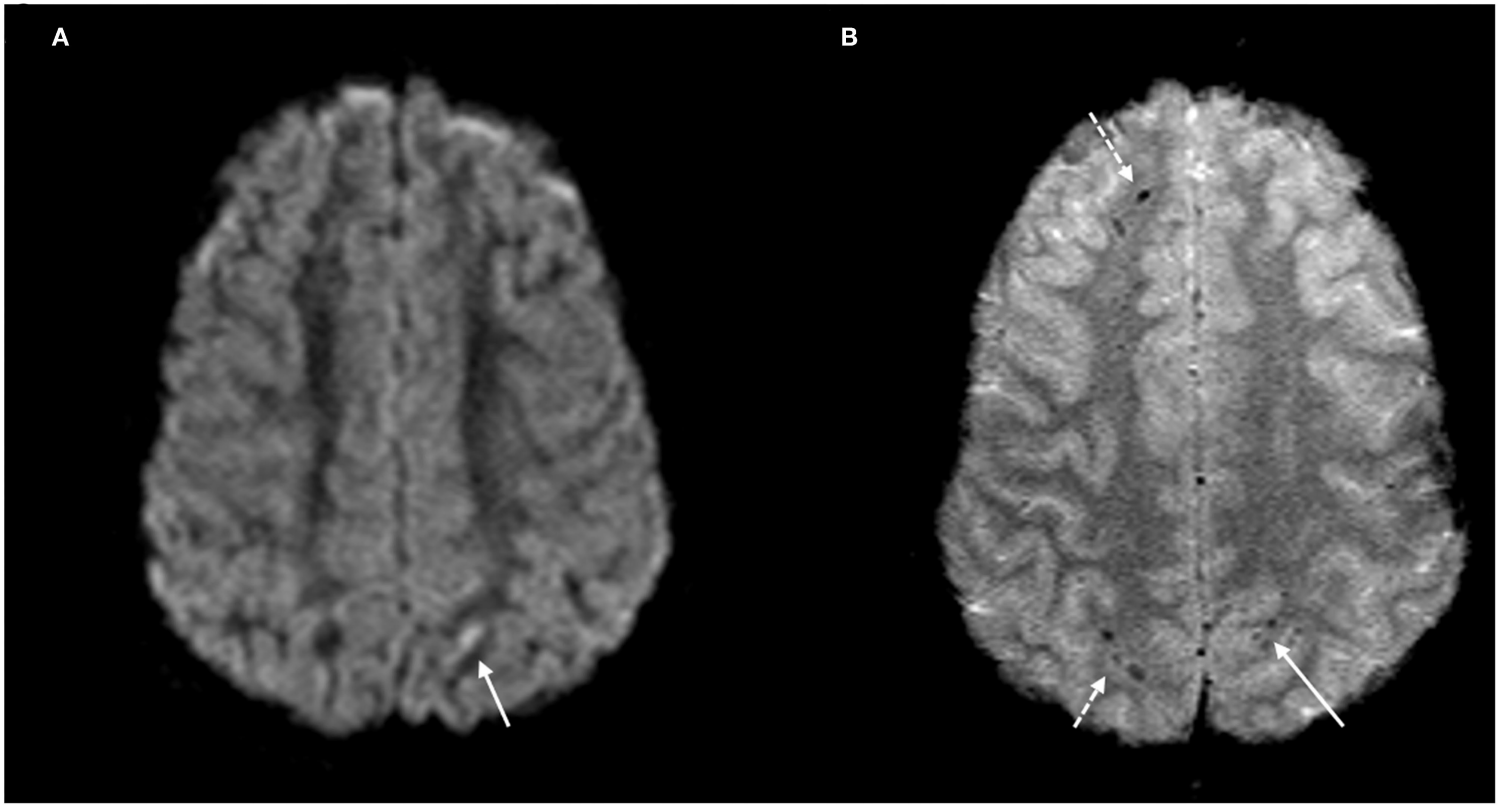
DAI Zone 1 (Superficial) MRI Findings. (A) axial diffusion weighted (DWI) and (B) axial T2^*^ weighted sequences show a punctate focus of restricted diffusion (A, white arrow) at the left parietal gray-white junction with associated susceptibility effect (B, solid white arrow). Additional foci of susceptibility within the frontal and parietal subcortical WM (B, broken white arrows).
